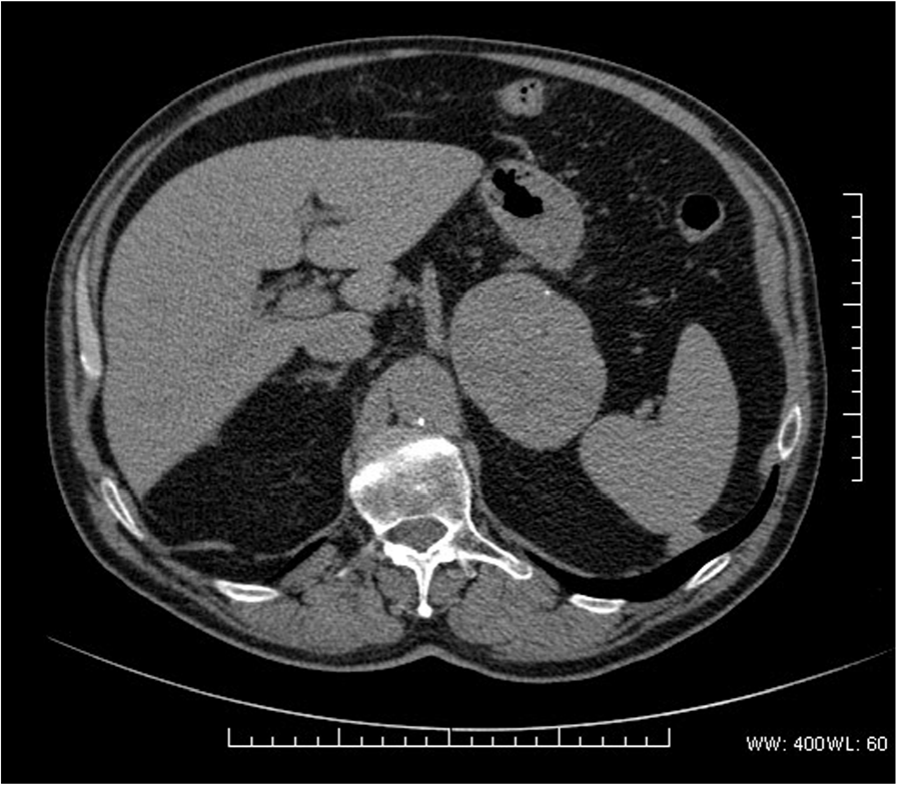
CT scan showing a large homogeneous lesion with rare peripheral calcifications
